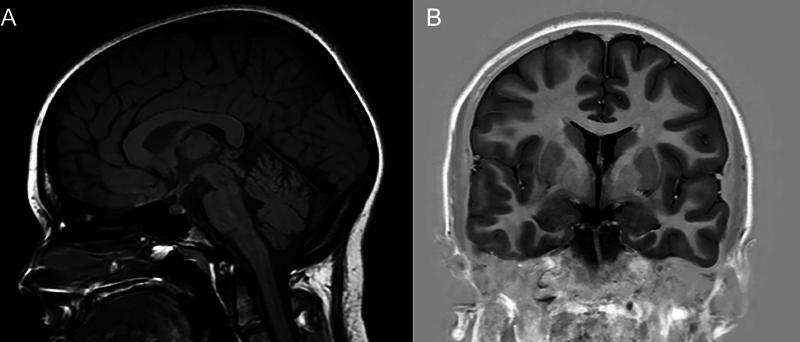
Githa was born by emergency cesarean at 42 weeks gestational age, birth weight 3,490 grams, and a poor start (Apgar 1/1) due to a uterine rupture. Umbilical cord pH was 6.75. Brain MRI at 10 days after birth was classified as normal. At 24 months, her neurodevelopment was normal according to the Griffiths Mental Developmental Scales (GMDS). At 6 years of age, her mother reported concerns about her short-term memory. No abnormalities were noted on brain MRI at 7 years of age. Brain MRI was repeated at 10 years of age, and mild abnormalities in the hippocampi were noted. Brain MRI was performed again at 17 years. In addition to mild abnormalities in the hippocampi, in retrospect, mammillary body atrophy was noted on the sagittal T1-weighted (
